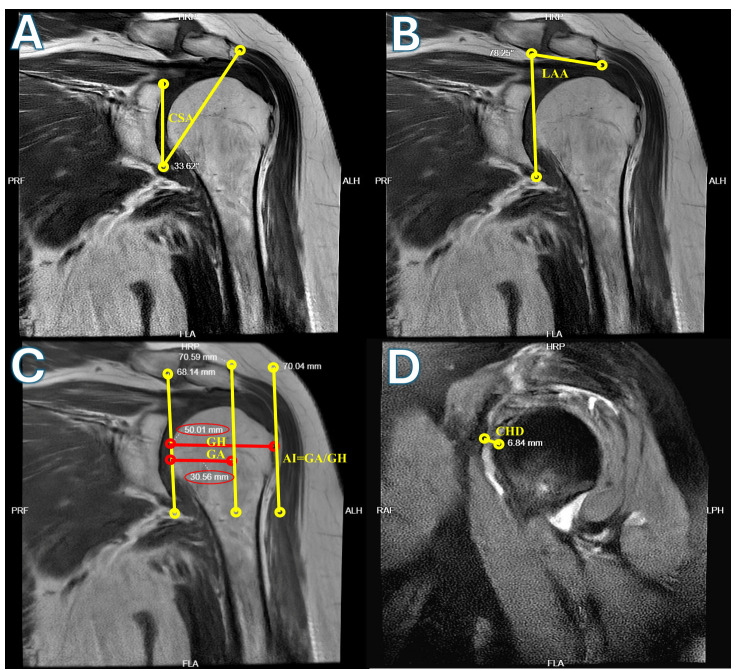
Angular and morphometric measurements on shoulder MRI. (A) Critical shoulder angle (CSA), measured as the angle formed between the glenoid line and the line connecting the inferior glenoid margin to the lateral edge of the acromion. (B) Lateral acromial angle (LAA), measured as the angle between the glenoid plane and the inferior surface of the acromion. (C) Acromial index (AI), calculated as the ratio of the lateral extension of the acromion to the lateral aspect of the humeral head, and acromiohumeral distance (AHD), defined as the shortest distance between the inferior surface of the acromion and the humeral head. Both measurements were obtained on a coronal oblique T1-weighted MRI image. (D) Coracohumeral distance (CHD), measured as the shortest distance between the coracoid process and the lesser tuberosity on an axial MRI image. HLP, ALH, PRF, and FLA represent orientation markers automatically generated by the MRI system.
